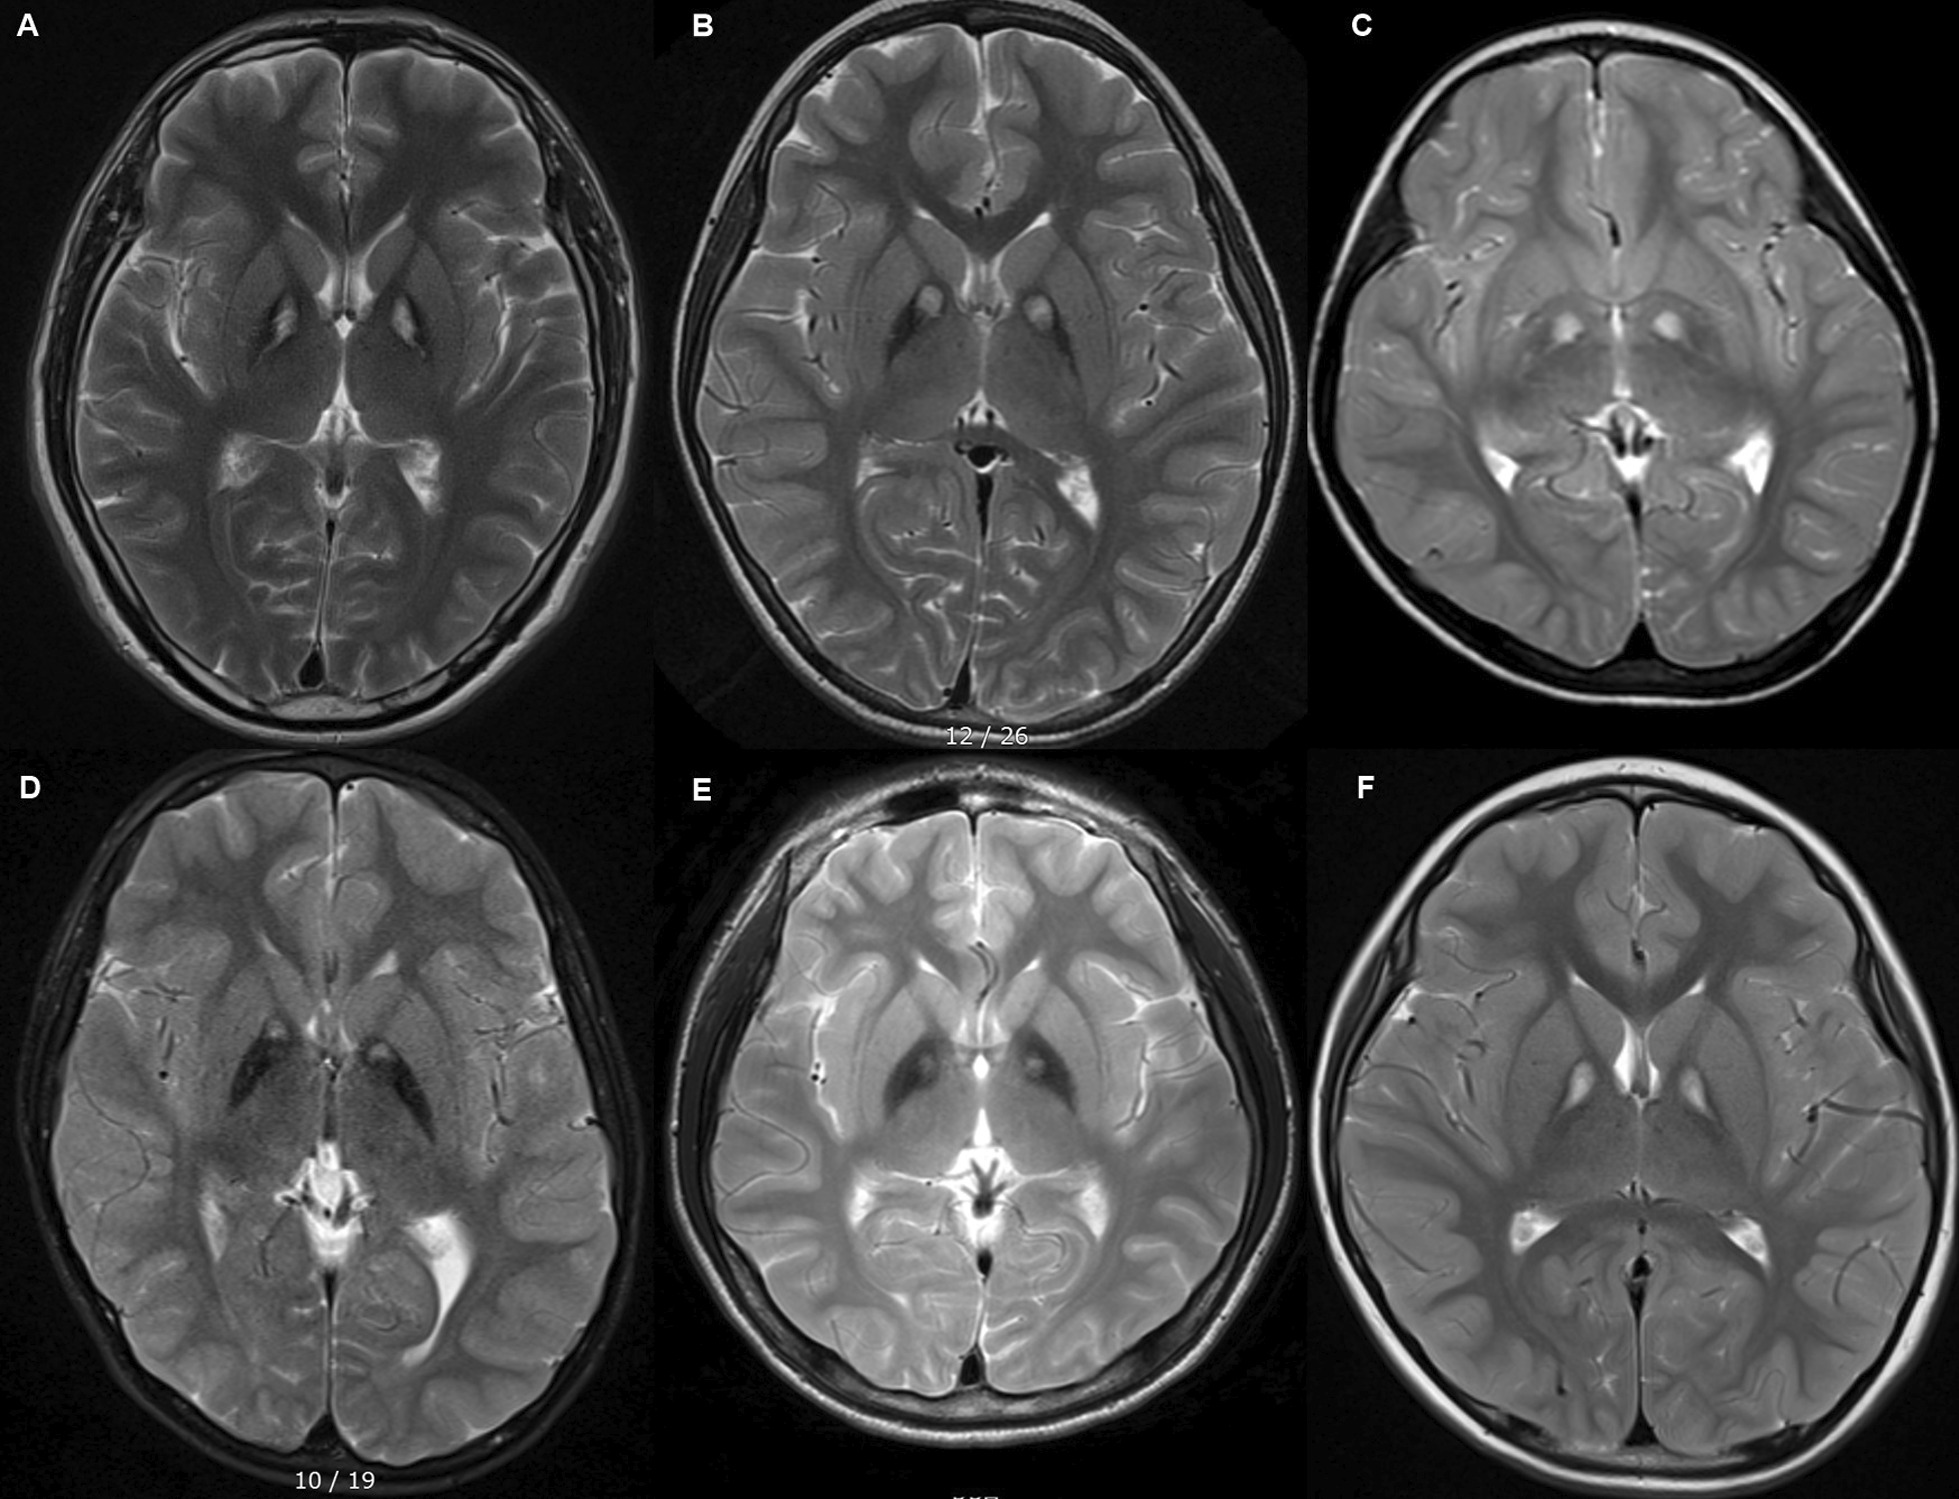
MRI T2 image of A P1, B P3, C P4, D P5, E P6, F P7 showing symmetrical central hyperintensity surrounded by hypointensity in the globus pallidus giving “eye’s of tiger sign” appearance
